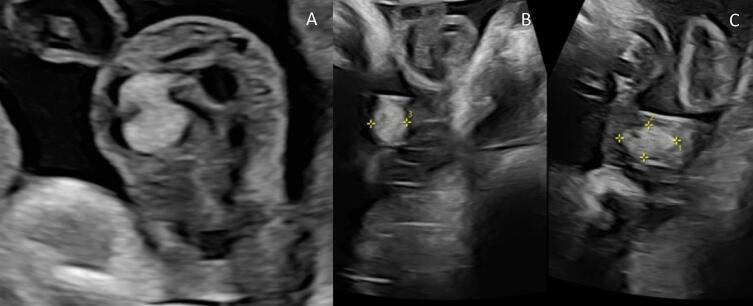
Ultrasound images demonstrating an echogenic mass within the umbilical cord, consistent with an umbilical cord hemangioma.
